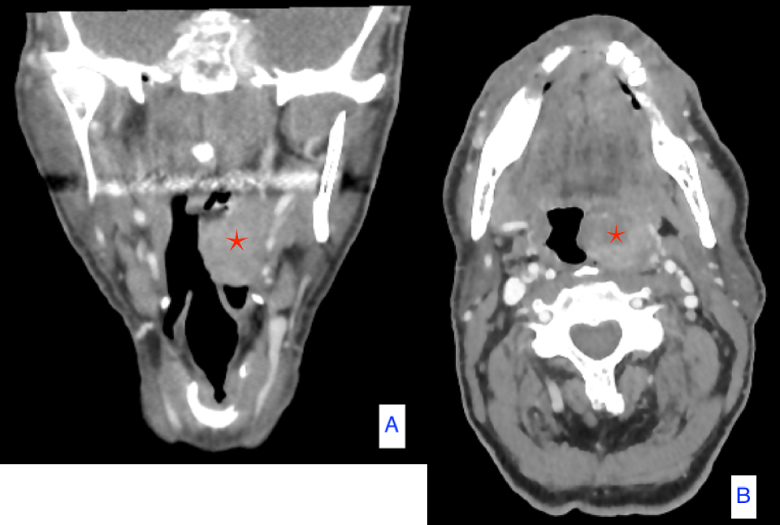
Computed tomography scans with contrast of the tonsillar mass (red asterisk) that occupies the left side of oropharynx. (For interpretation of the references to colour in this figure legend, the reader is referred to the web version of this article.)
